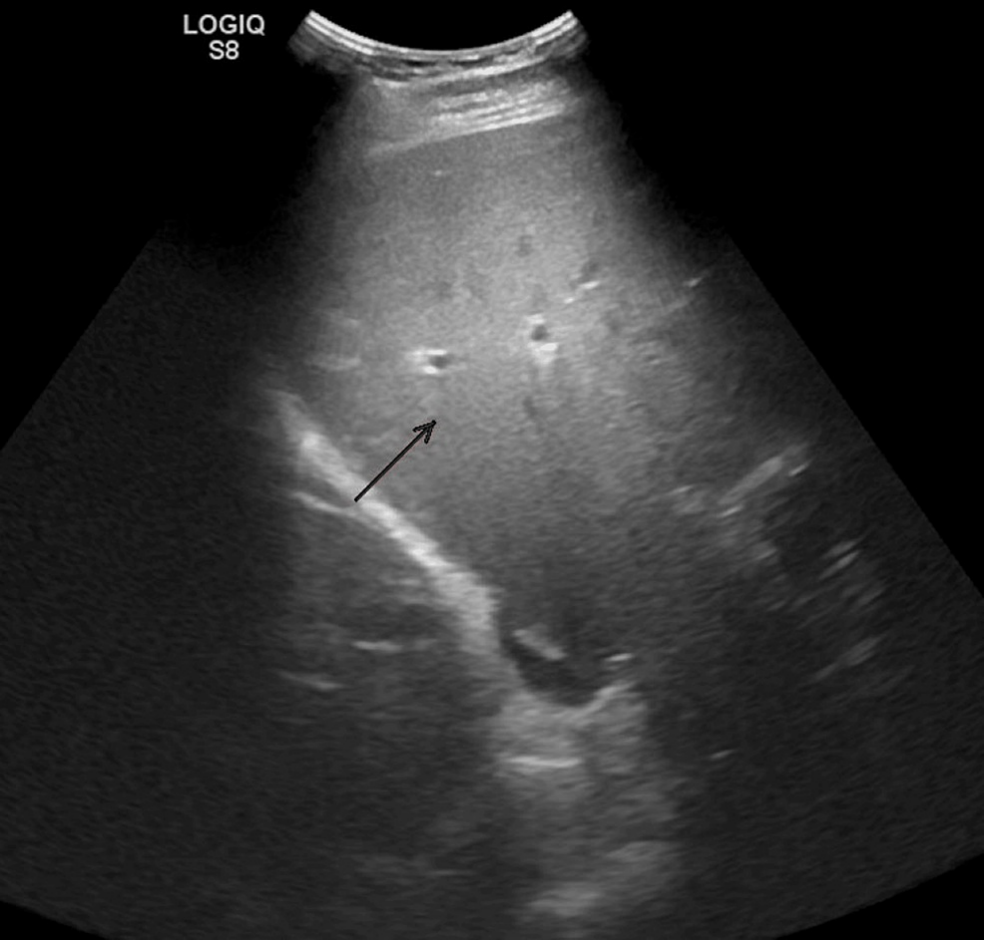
Ultrasound of the liver showing a heterogenous liver parenchyma with no intrahepatic or extrahepatic biliary ductal dilatation.
